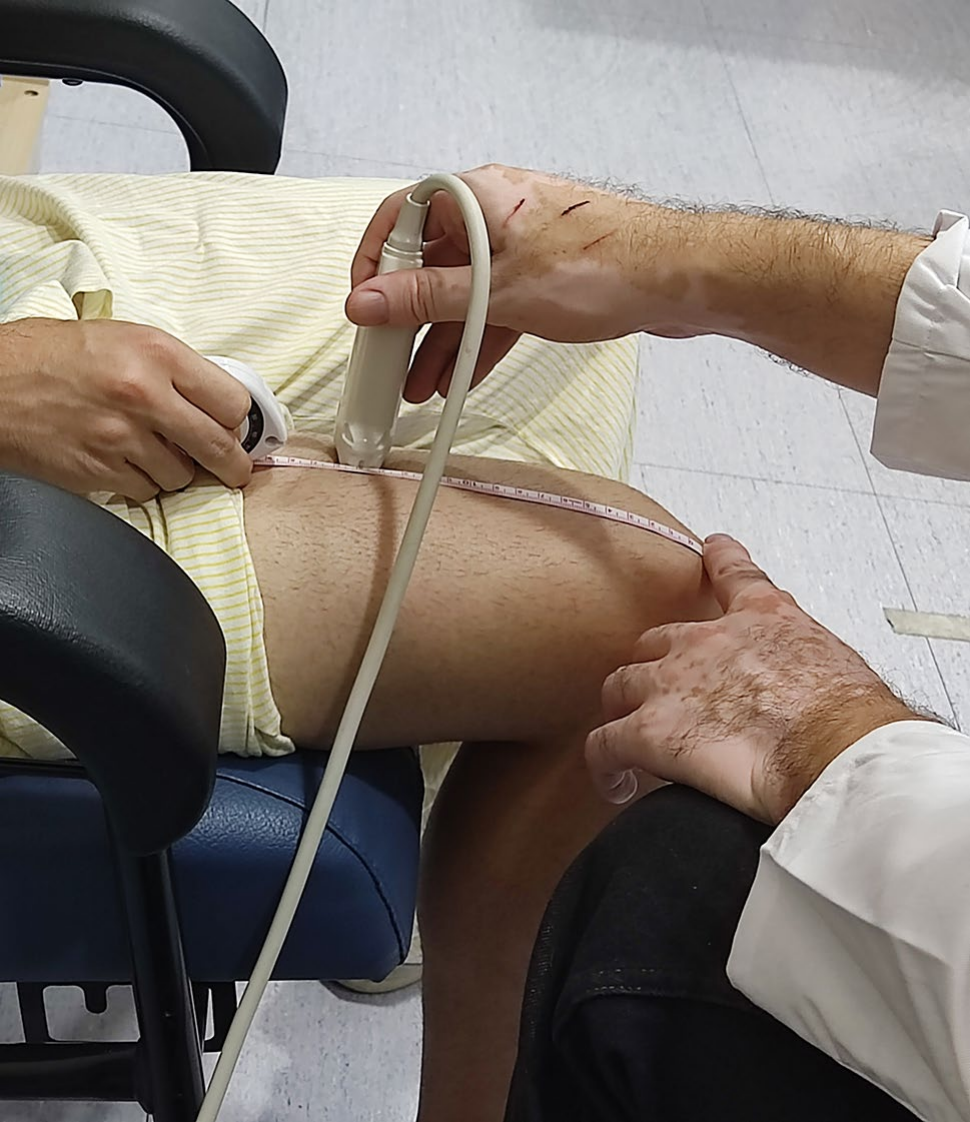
The landmark and the patients´ position for the ultrasound assessment
